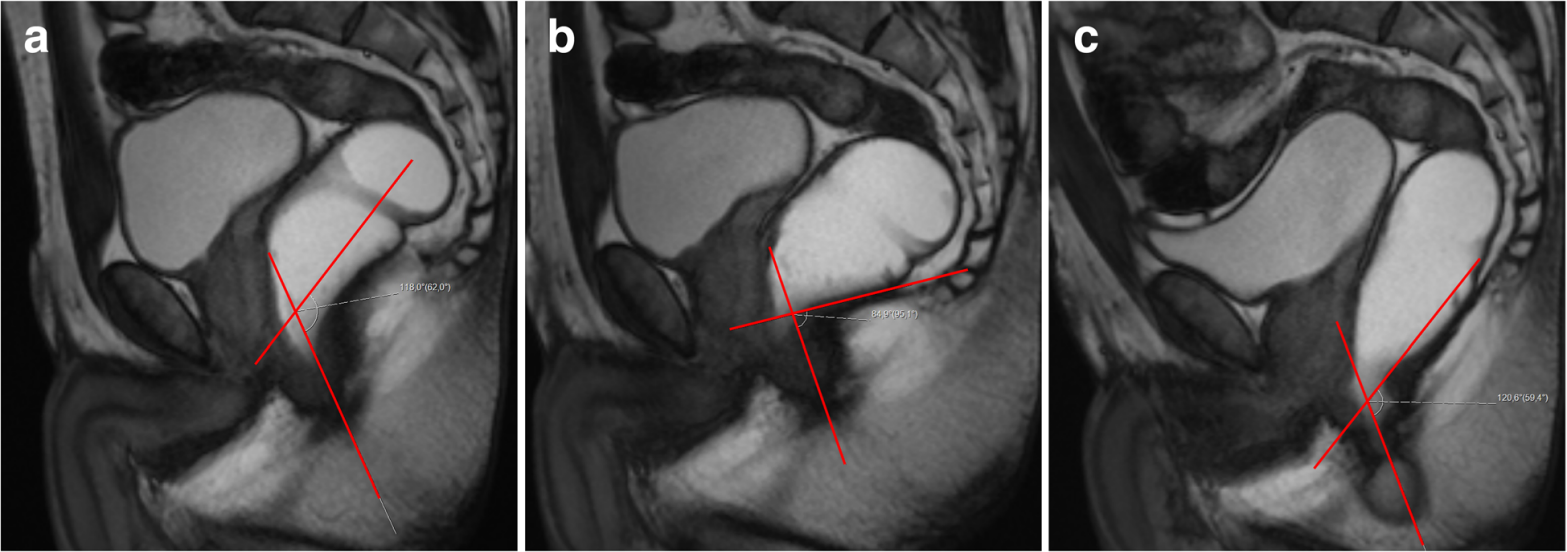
Spastic pelvic floor syndrome. (a, b, c) Sagittal midline TrueFISP images of a man at rest (a), during squeezing (b) and evacuation (c), demonstrating a normal ARA angle at rest in (a) (118°), but revealing an increased reduction during squeezing in (b) (85°) and a lack of normal widening in the evacuation phase (c) (120°). During the dynamic evaluation, spastic contractions of the puborectalis muscle during evacuation were noted as well, and an incomplete evacuation with retention of ultrasound gel was present, all signs compatible with paradoxical contraction of the puborectalis muscle
